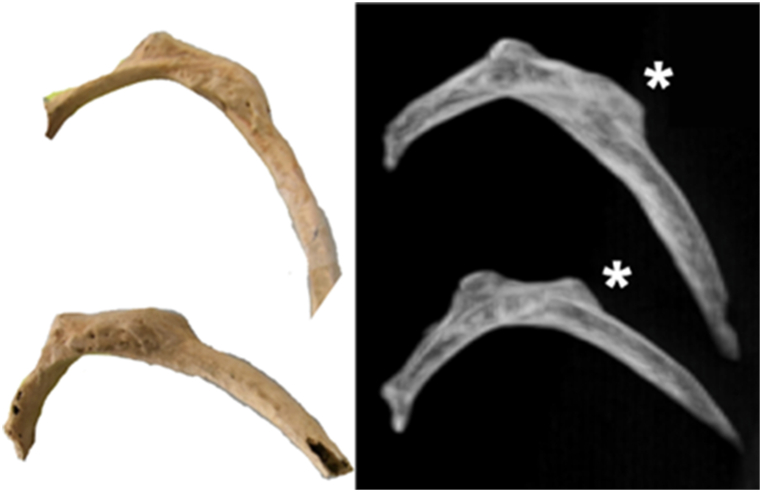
Photography and CT scan, MIP reconstruction (maximum intensity projection). Left 4th and 5th rib fractures, posterior aspect (asterisk).
